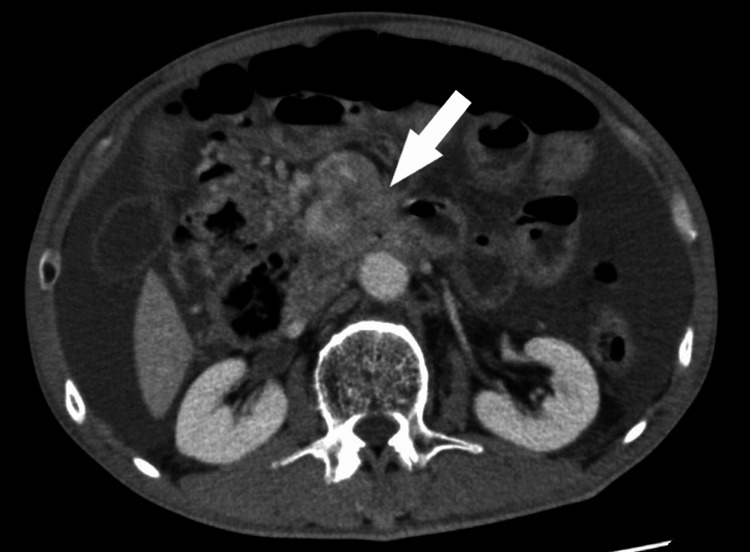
Abdominal contrast-enhanced CT scan (axial view). The tumor with delayed enhancement was confirmed in the pancreatic uncinate process (white arrow). CT: computed tomography
